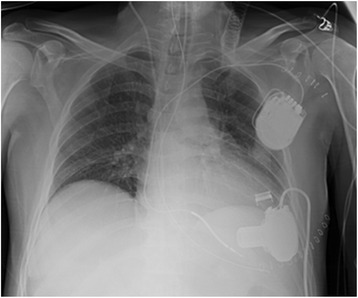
Chest X-ray of the multiorgan donor with an implanted LVAD one day before the donation
